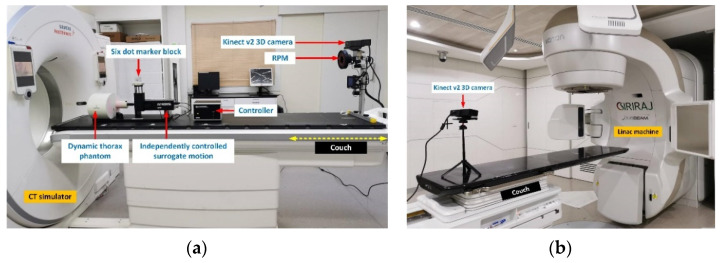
(a) Equipment setup used to collect datasets for training and testing the proposed time-series deep-learning models, and (b) the medical linear accelerator (LINAC) used to deliver high-energy X-rays for cancer treatment.
